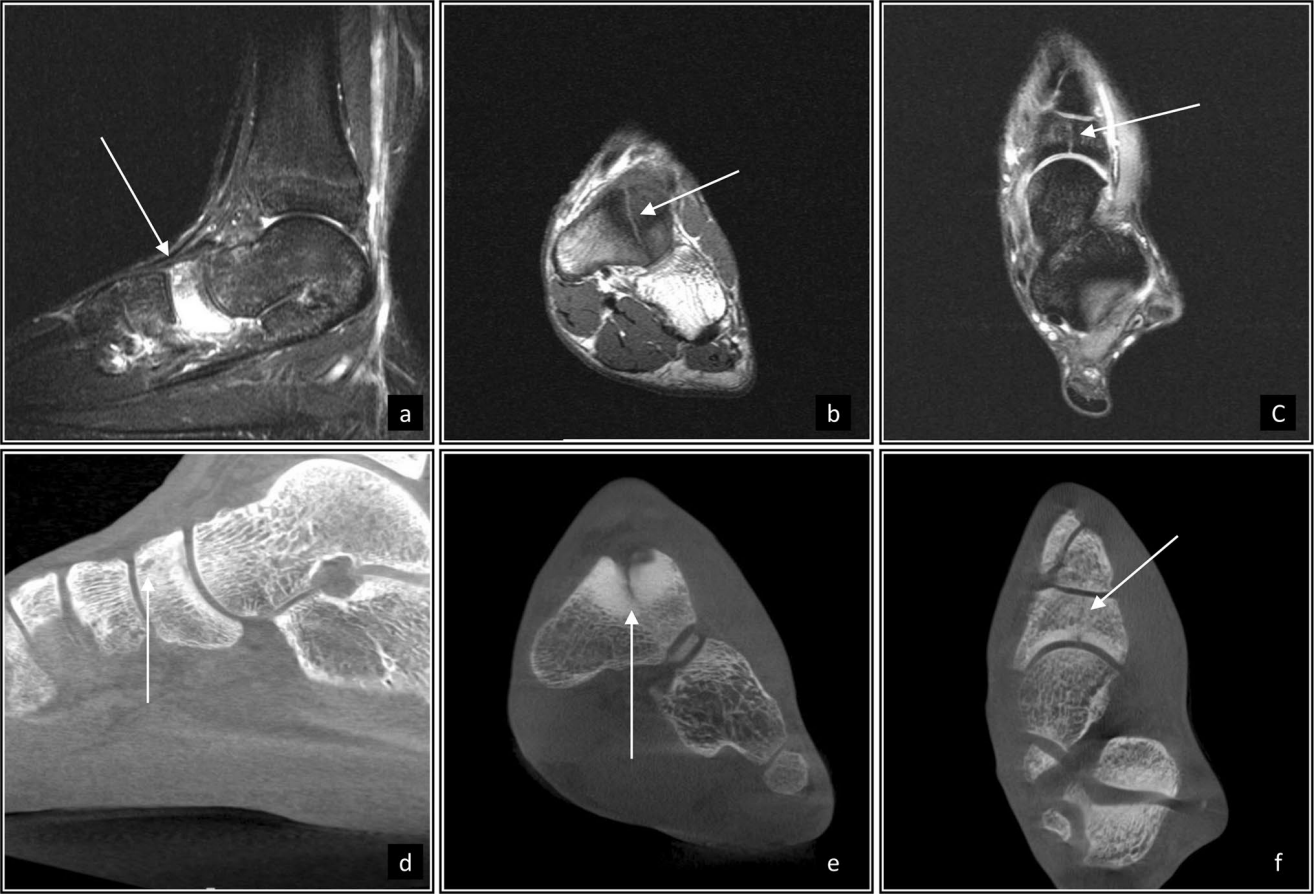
Images from Case 3. Long axis (a), sagittal (b), and coronal (c) on T2 fat-suppressed sequences on magnetic resonance imaging demonstrate vertically oriented stress fracture. Corresponding views on CT visualize the fracture orientation (d–f) extending through both plantar and dorsal cortices and classify as navicular Type III stress fracture [20]
